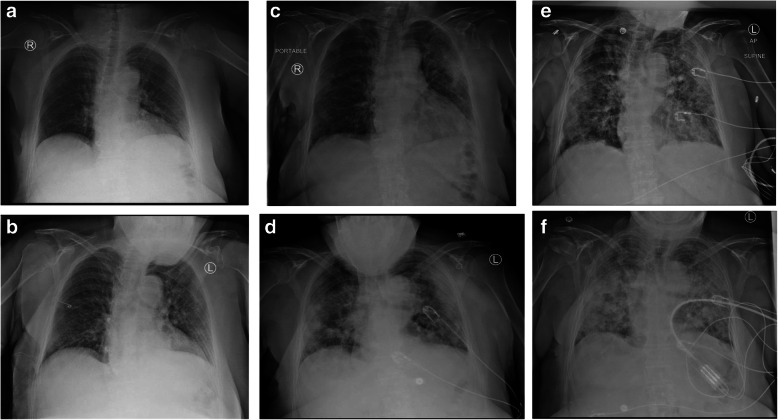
Series chest x-rays in an 80-year-old woman with COVID-19 pneumonia. a Chest x-ray obtained on illness day 5 showed peripheral GGO in the LLZ (score 1). b Chest x-ray obtained on illness day 7 showed increase extent of the GGO diffusely involving the left lung (score 4). c Chest x-ray obtained on illness day 11 showed increase extent of the GGO involving the right lung, with increase extent of consolidation involving the left lung diffusely (Total score 8). d Chest x-ray obtained on illness day 14 showed development of reticulations in both lungs with increase extent of involvement of the RUZ. (Total score 8). e Chest x-ray obtained on illness day 17 showed extensive bilateral consolidations mainly peripherally with increased reticulations (Total score 8). f Chest x-ray obtained on illness day 18 showed extensive consolidation involving both lungs diffusely (Total score 8). The patient died on illness day 18. (GGO: ground glass opacity. LLZ: left lower zone. RUZ: right upper zone)
